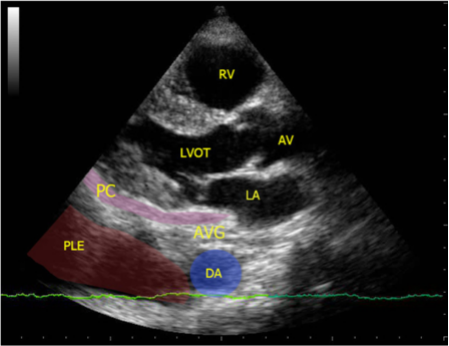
Pleural effusion (*PLE*). The parasternal long axis view shows anechoic pleural effusion (*PLE*) accumulated posterior to the descending aorta (*DA*). Pericardium (*PC*) is represented by the *pink strip* here. Also pleural effusion, if large amount, can extend beyond the atrioventricular groove in contrast with pericardial effusion which terminates at the atrioventricular groove. (*AV* aortic valve, *LVOT* left ventricular outflow tract, *PC* pericardium, *PLE* pleural effusion, *RA* right atrium, *RV* right ventricle, *DA* descending aorta, *AVG* atrioventricular groove)
